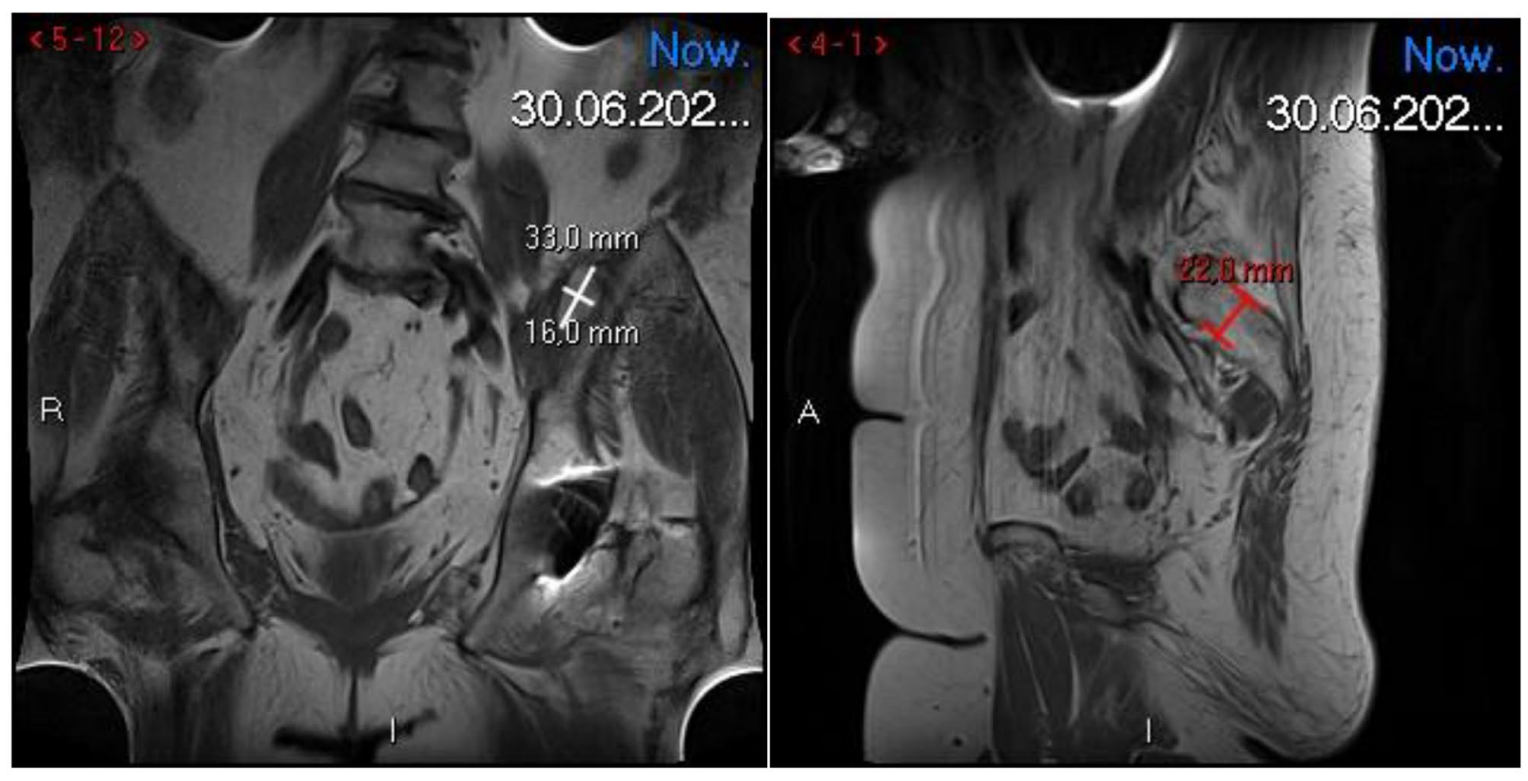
Control MRI with regression of hematoma
